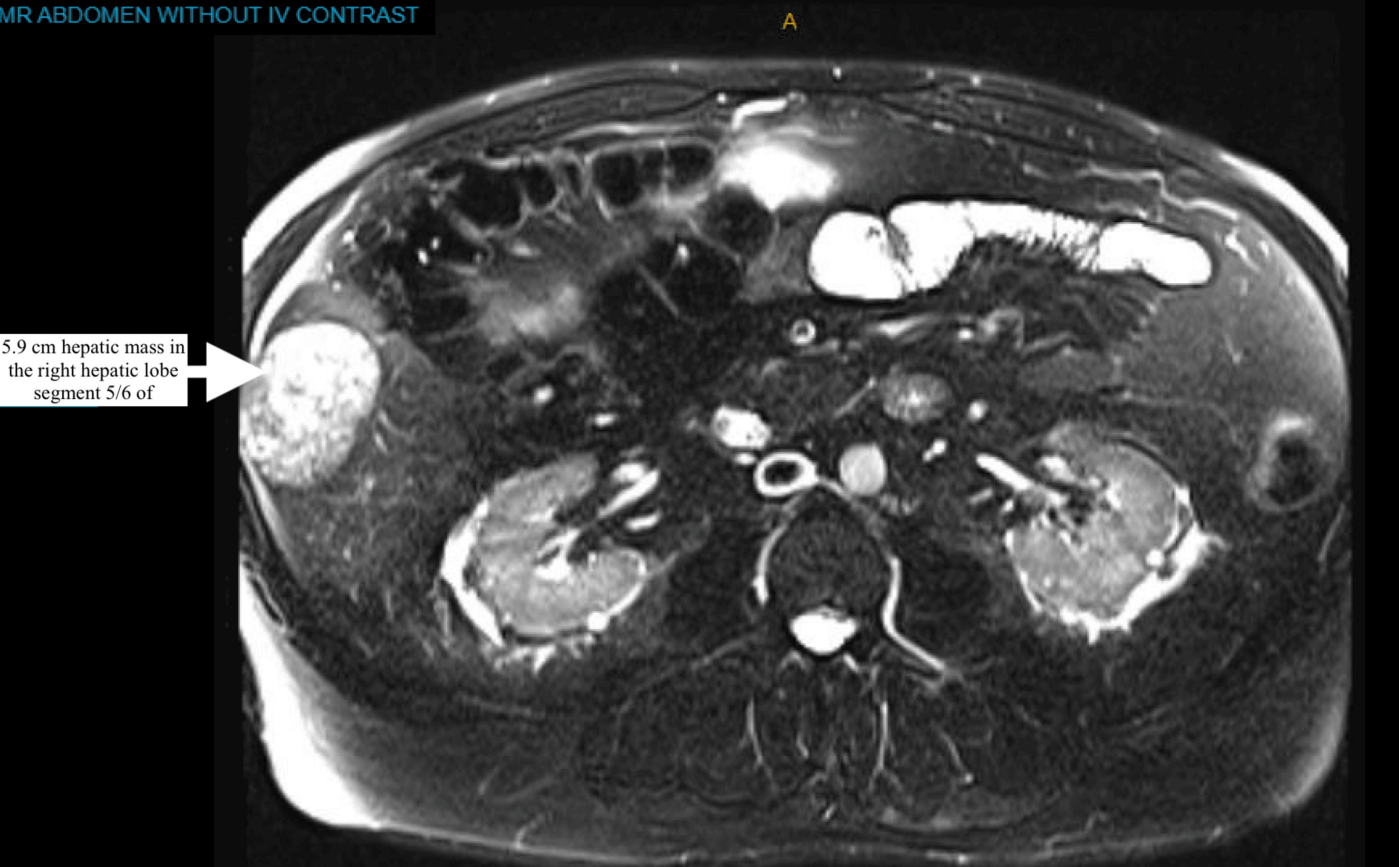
MRI of the abdomen, with a white arrow showing an increase in mass size
